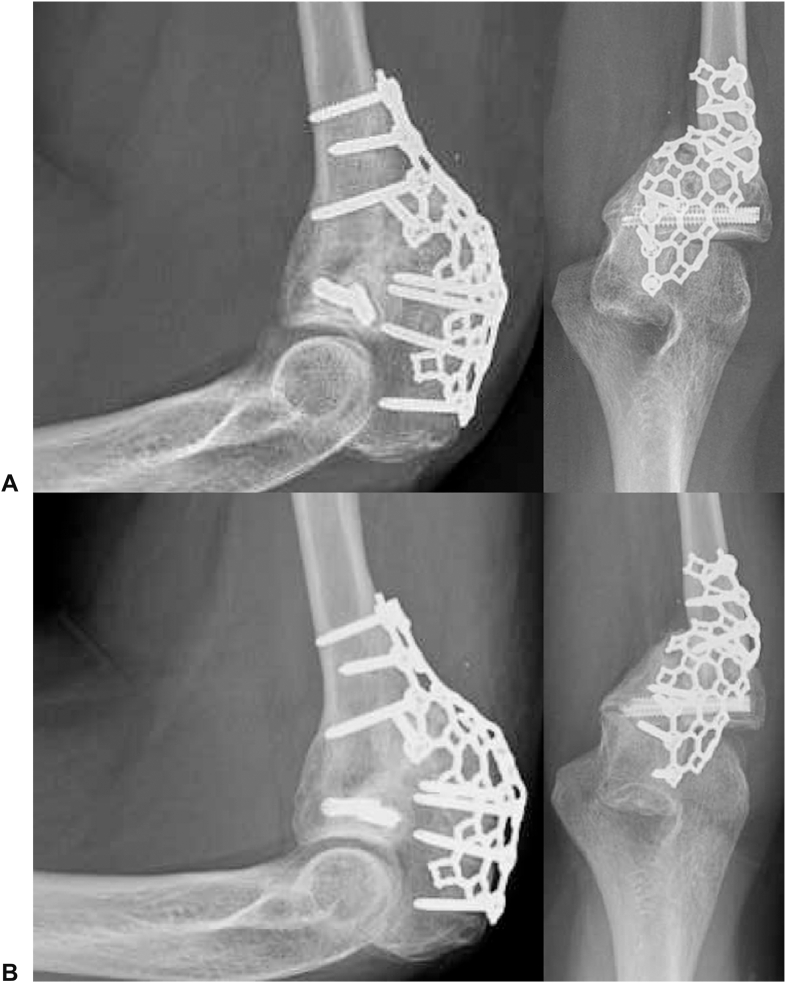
One-bone forearm reconstruction revision postoperative radiographs at A 10 weeks and B 18 months.
